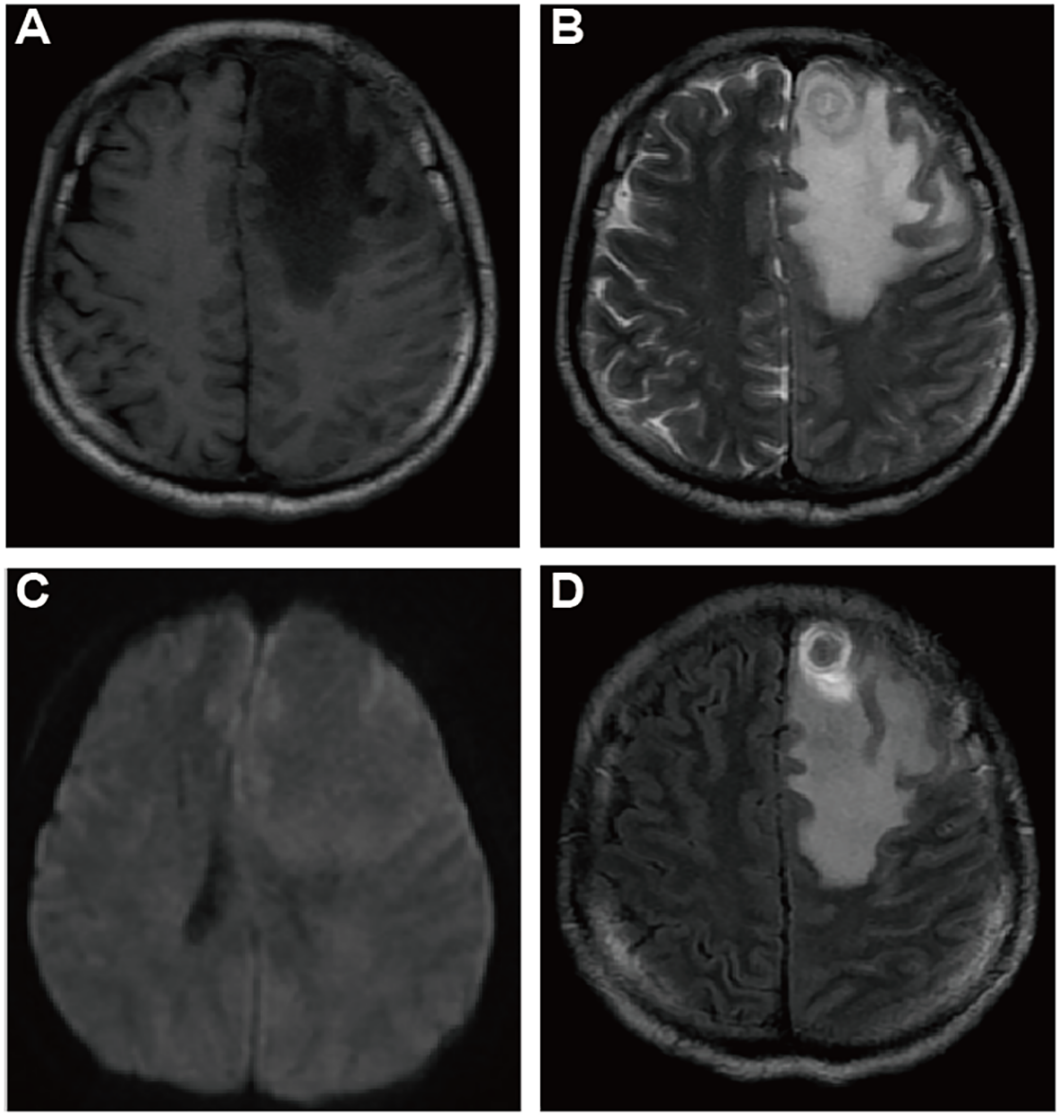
Cranial Magnetic Resonance Imaging (CMRI) of suspected brain metastases: CMRI T1WI (A), T2WI (B), DWI (C), Enhanced T1WI (D) axial images demonstrating a suspicious metastatic lesion: a subcortical roundish nodule located in the left frontal lobe, with low signal in T1WI and slightly hyperintense concentric ring pattern and uneven signal in T2WI, with peripheral circumferential ring-like enhancement, surrounded by a large area of edema, no apparent diffusion restriction.
